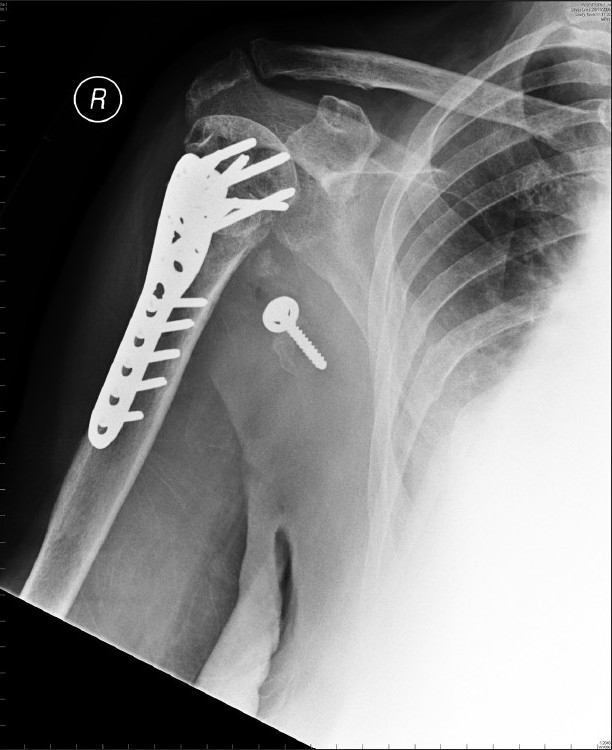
Antero-posterior radiograph at 1 year. Proximal humerus has healed. Coracoid has displaced
